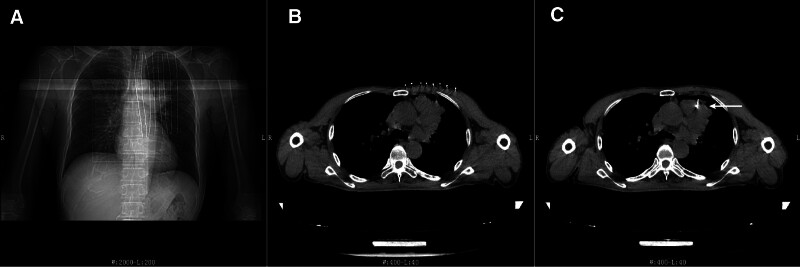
Puncture biopsy of the left lung using CT-guided localization for space-occupying lesion. The operator attaches a metal positioning wire to the patient body surface (A) and then uses tomographic images from the CT scan to determine the relative position of the left lung lesion to the body surface metal positioning wire, further planning the precise puncture path (B). Employing a 20G puncture biopsy needle (←), left lung lesions were biopsied under CT guidance (C). CT = computed tomography.
